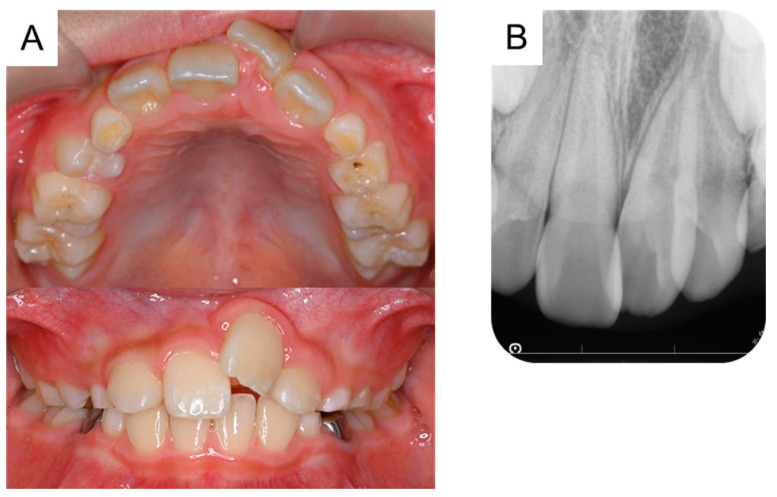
Intraoral photographs at regular checkups. (A) Intraoral photograph and (B) periapical radiograph obtained in the postoperative period at the age of 9 years and 7 months.
